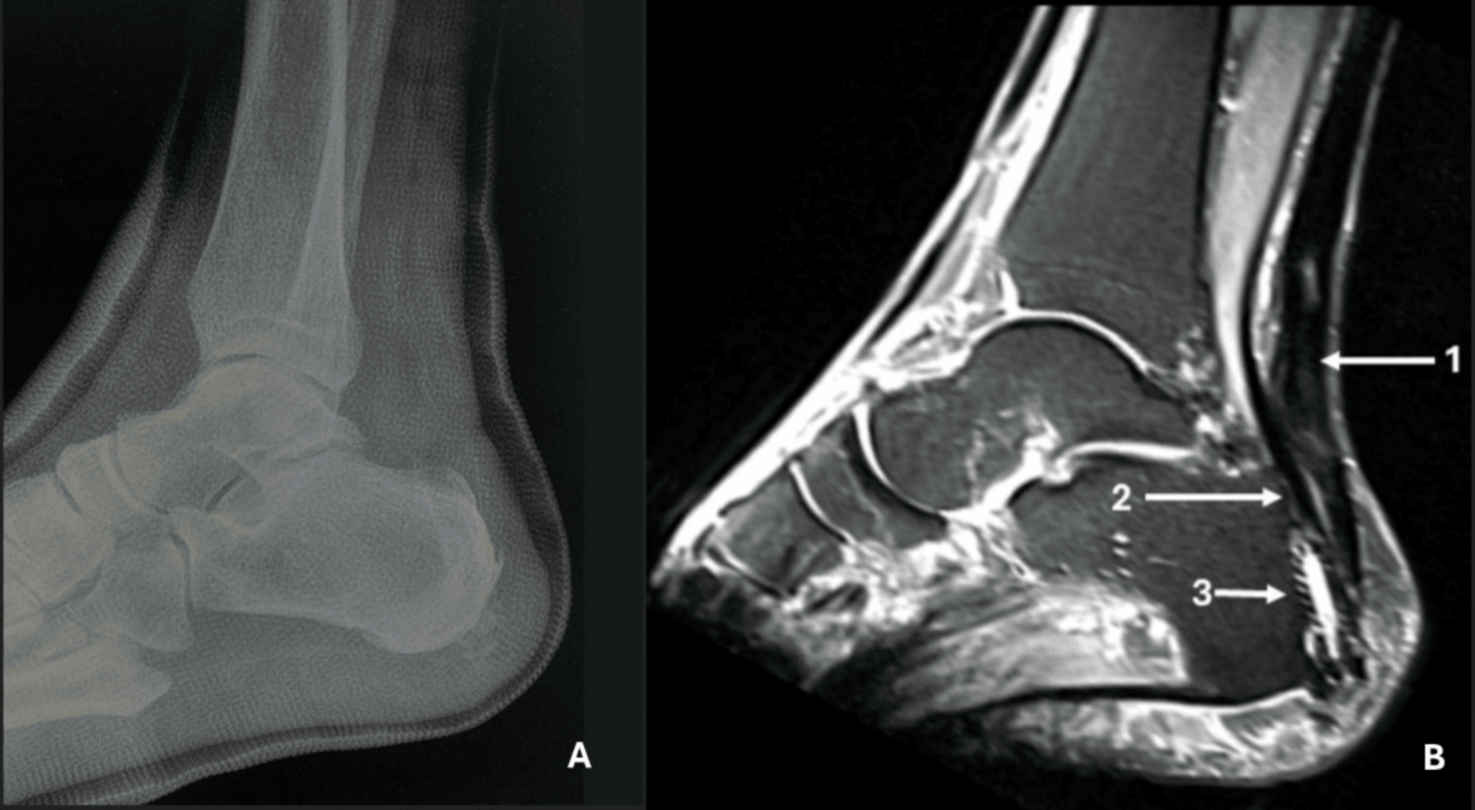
(A) Postoperative lateral ankle radiograph. (B) Postoperative T2 MRI at 24-month follow-up. Arrow 1 indicates the homogenous signal of the healed Achilles tendon. Arrow 2 depicts the transferred FHL tendon. Arrow 3 shows the fixation of the FHL with the bioabsorbable interference screw. MRI: Magnetic Resonance Imaging, FHL: Flexor Hallucis Longus
